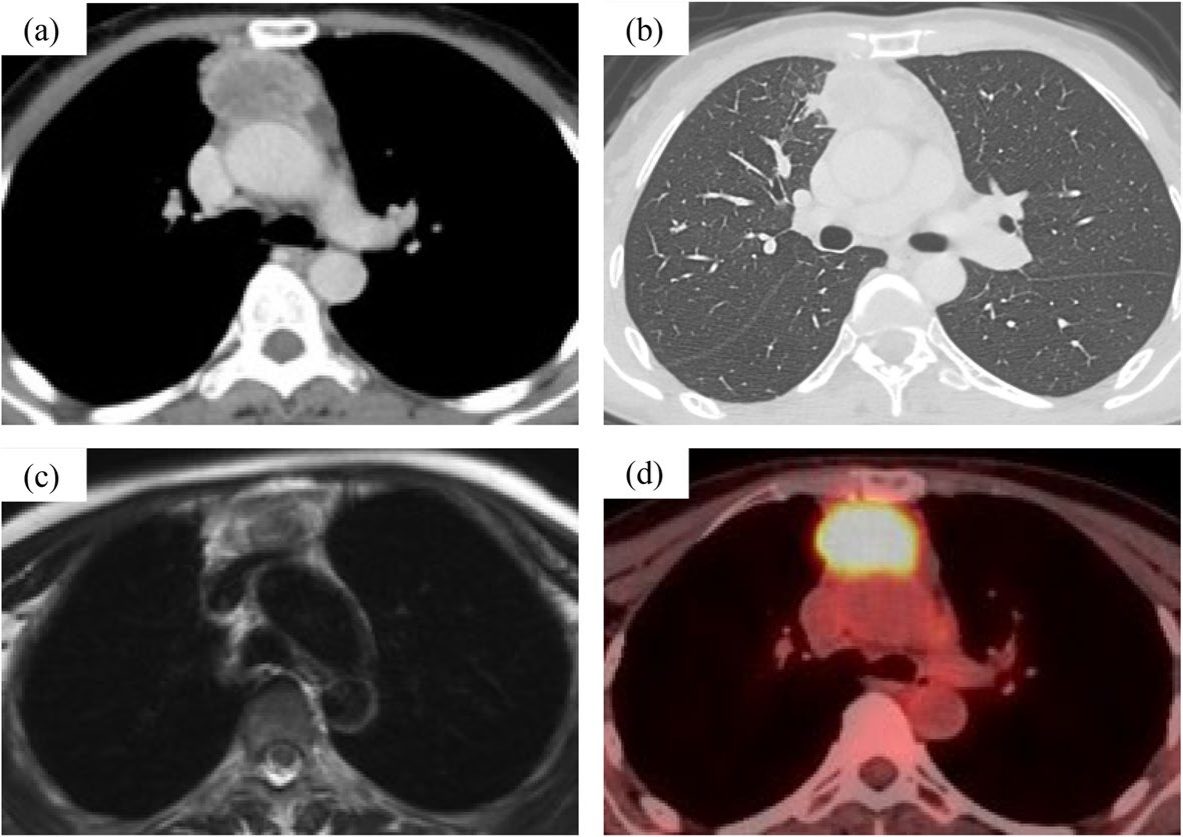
Radiological findings of the tumor (a) Chest computed tomography displays a 5.5-cm diameter mass in the anterior mediastinum. b The tumor directly invades the right upper lobe. c Magnetic resonance imaging reveals no obvious vascular invasion. d Fluorodeoxyglucose-positron emission tomography shows abnormal uptake in the mediastinal mass
